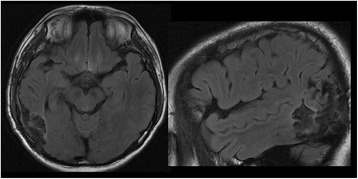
Postsurgical MRI of Case 1 and seizure freedom has lasted for 25 months since cortical resection
